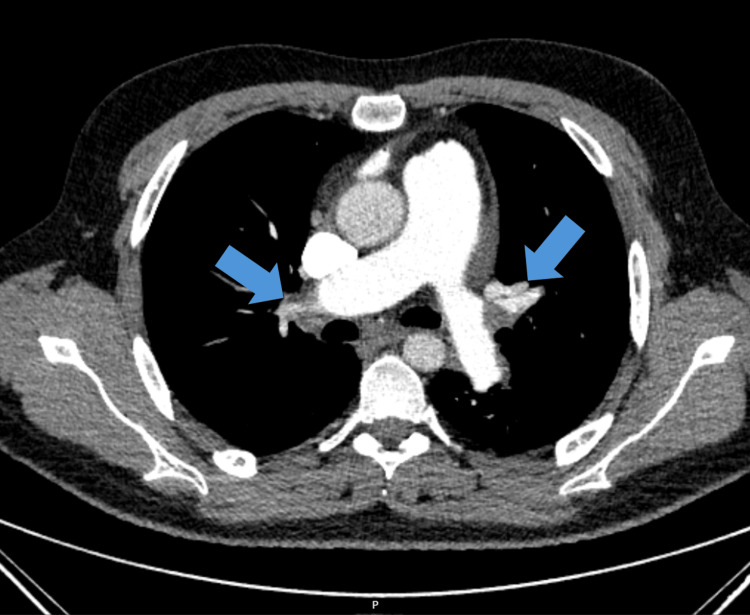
Thoracic CTA in the emergency room showing the filling defects (blue arrows) in the right pulmonary artery and a branch of the left pulmonary artery CTA: computed tomography angiography
